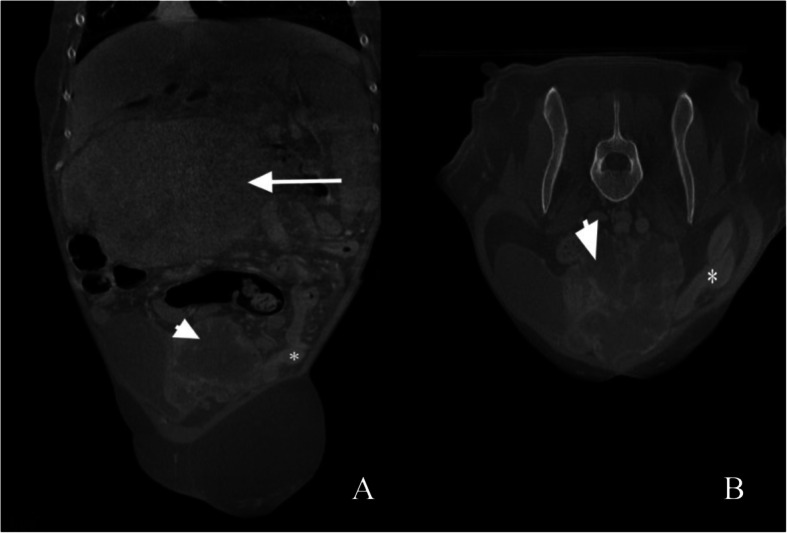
Dorsal (a) and transverse (b) reconstructed postcontrast, soft tissue algorithm, computed tomography images of the abdomen. Hydroureter (*) secondary to an expansile obstructive mass (short arrow) of the left ureter is visible as well as an attenuating mass of the left lateral liver lobe (long arrow)
